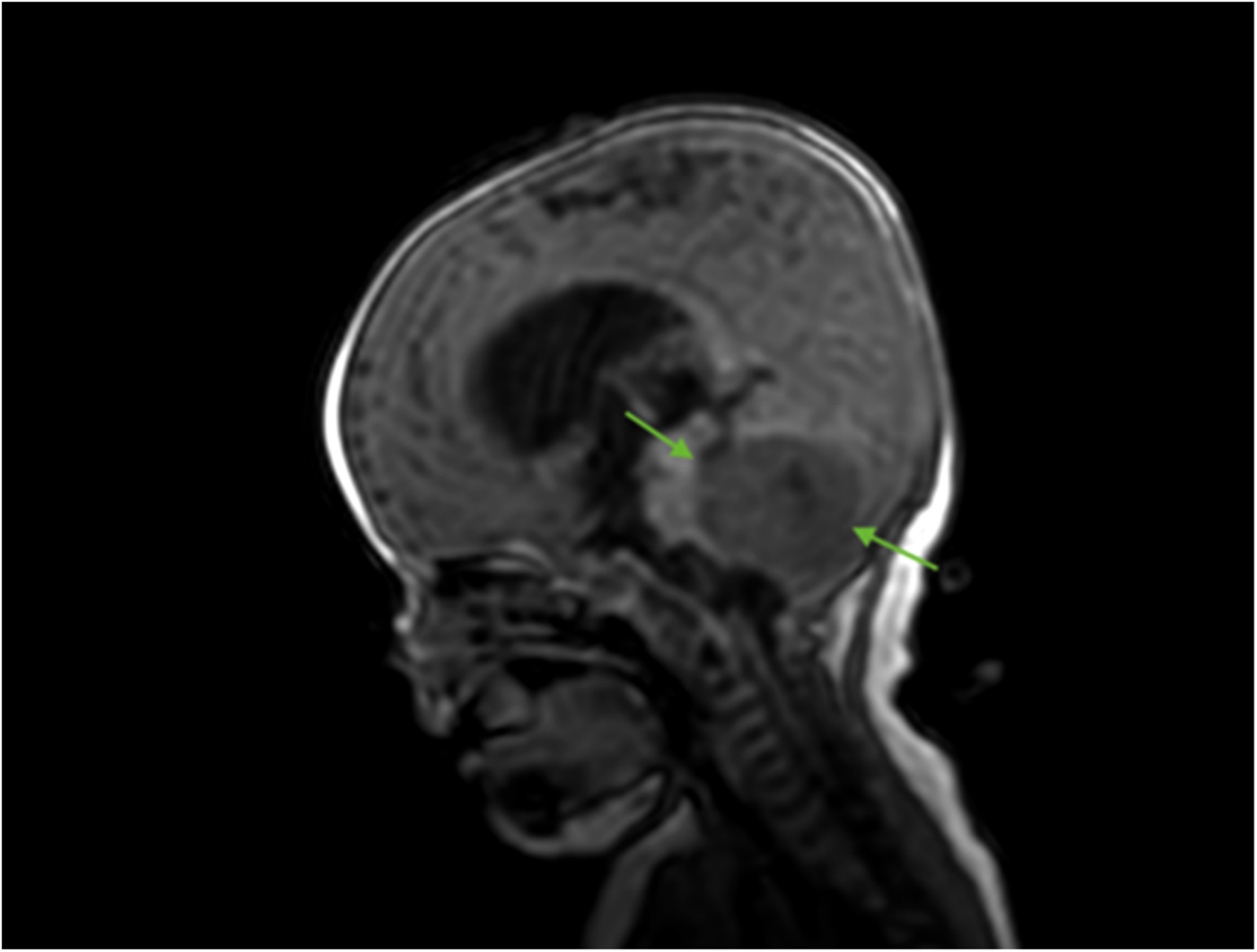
Brain MRI (sagittal T2 + C). Preoperative MRI shows a posterior fossa tumor (green arrows).
